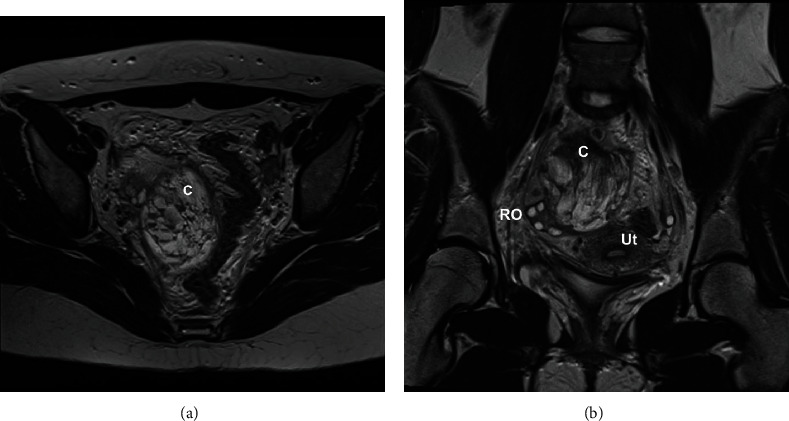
MRI of the pelvis. (a) (11) Axial T2-weighted image demonstrating the large complex cystic mass (C) located at the right hemipelvis. The lesion is multiloculated with internal haemorrhage seen as fluid-fluid levels and T2 shading (darker cyst content). (b) Coronal T2-weighted image with the normal right ovary (RO) is demonstrated lateral to the complex cystic mass (C). Large irregular low T2 signal intensity regions within the cystic lesion relate to blood products. Ut: uterus.
